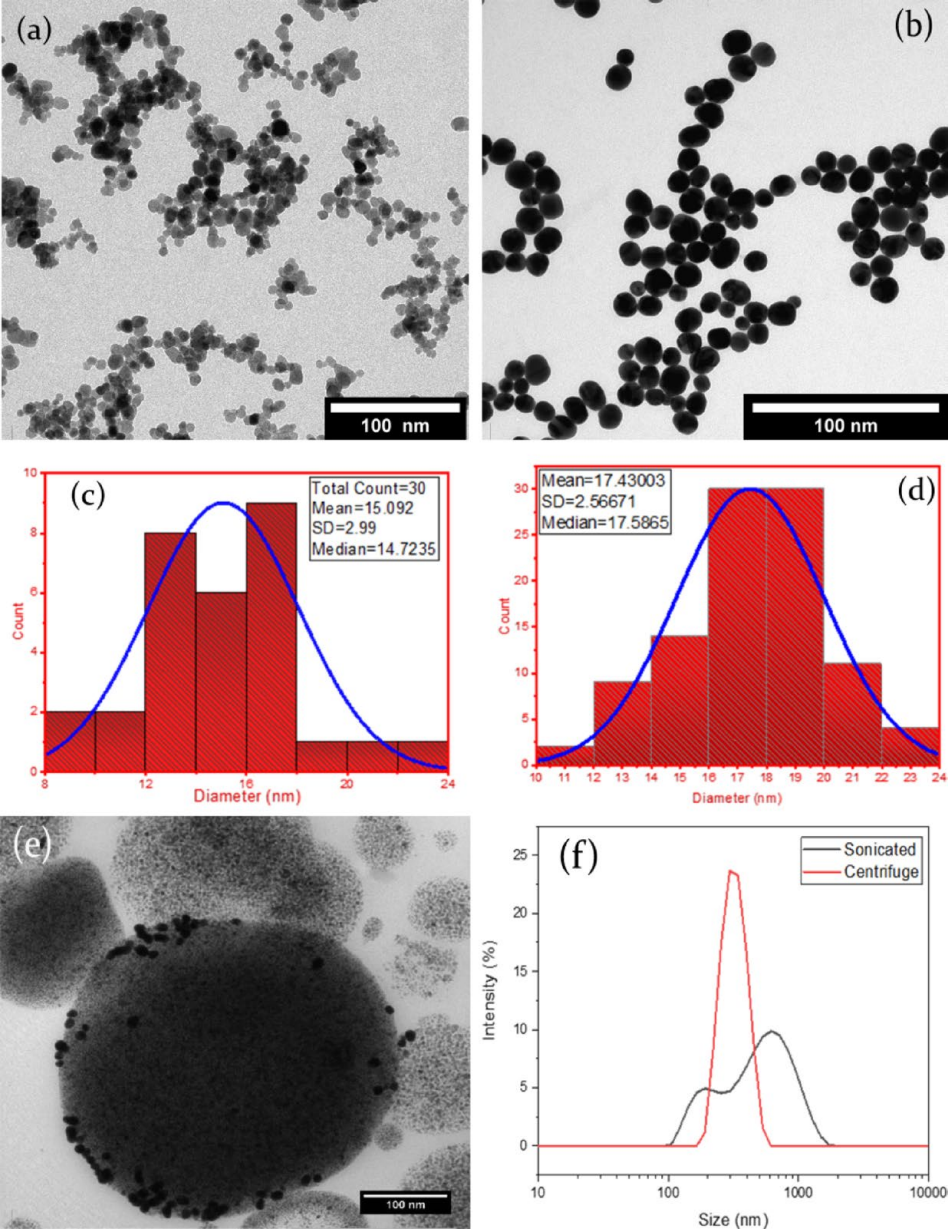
TEM images of: (a) Fe_3_O_4_ and (b) gold with their respective particle size distribution histogram (c & d), (e) Au pickering ferrofluid droplet (e) TEM images of Au pickering ferrofluid droplet. (f) Size distribution of ultrasonicated Au pickering ferrofluid emulsion averaging 600 ± 500 nm (Black line), and after centrifugation with distribution averaging 330 ± 200 nm. (a, b, c, d & f) are reproduced from Ref. 47 with permission from Hybrid adv., Elsevier, (e) is reproduced from Ref 46 with permission from the Royal Society of Chemistry).
